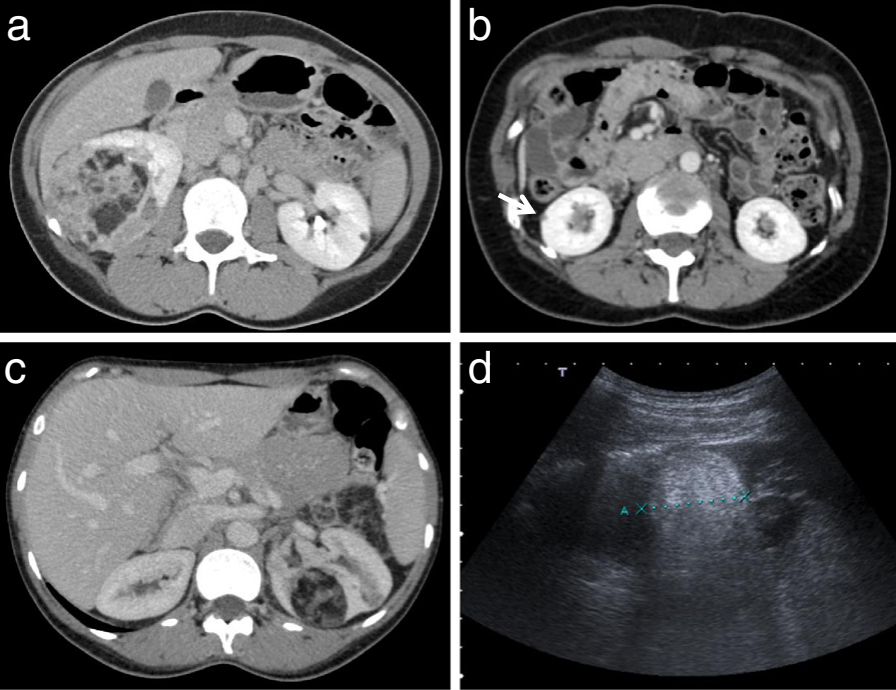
Imaging appearances of angiomyolipomas. (a) Characteristic CT appearance of large right renal angiomyolipoma showing a heterogenous lesion with areas of fat density and a small lesion in the contralateral kidney. (b) Tiny exophytic cortical angiomyolipoma in the right kidney assigned a measurement of 5 mm (arrow). (c) & (d) CT and ultrasound appearances respectively of the same 40 mm angiomyolipoma.
